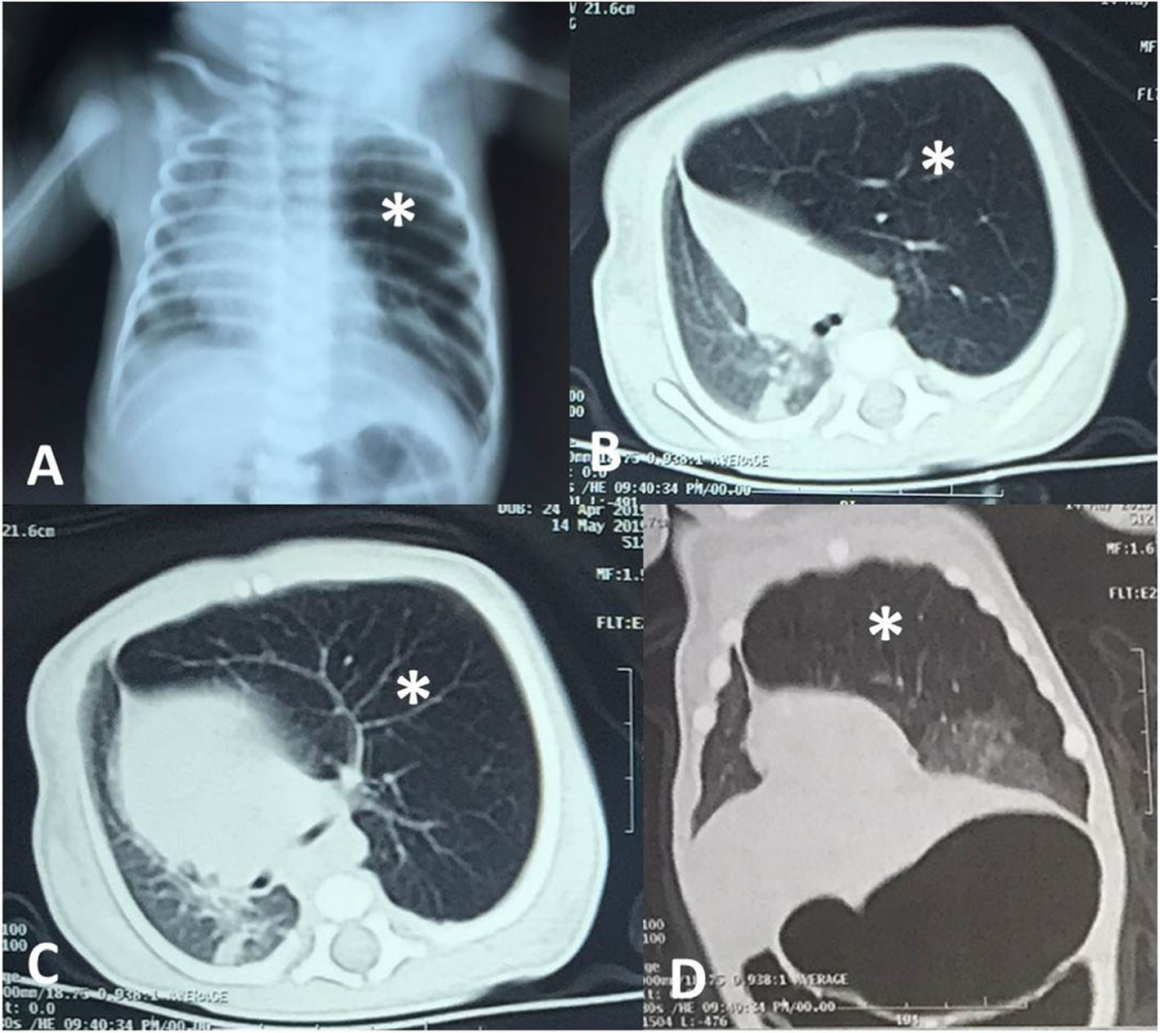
Male infant 5 weeks old has congenital left upper lobe over inflation. a Chest X-Ray, b&c Axial CT lung window and d Oblique coronal CT lung window showing the characteristic radiological findings of congenital lobar overinflation (*) and mediastinal shift. Crossing of the overinflated lobe to the other side is also noted
